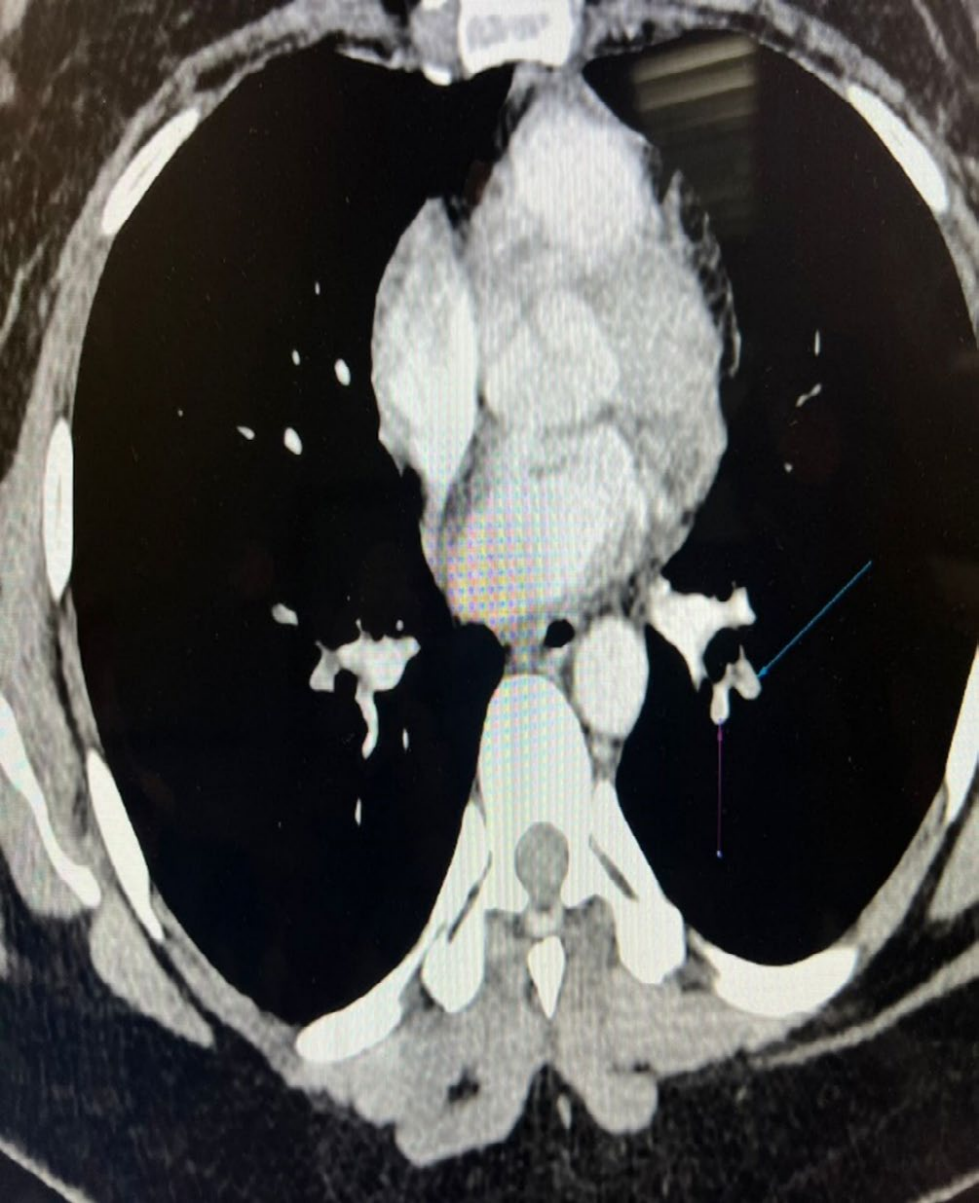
Shows a CT angiography of the pulmonary arteries, revealing a completely occluding hypodense thrombus in the left main pulmonary artery.
